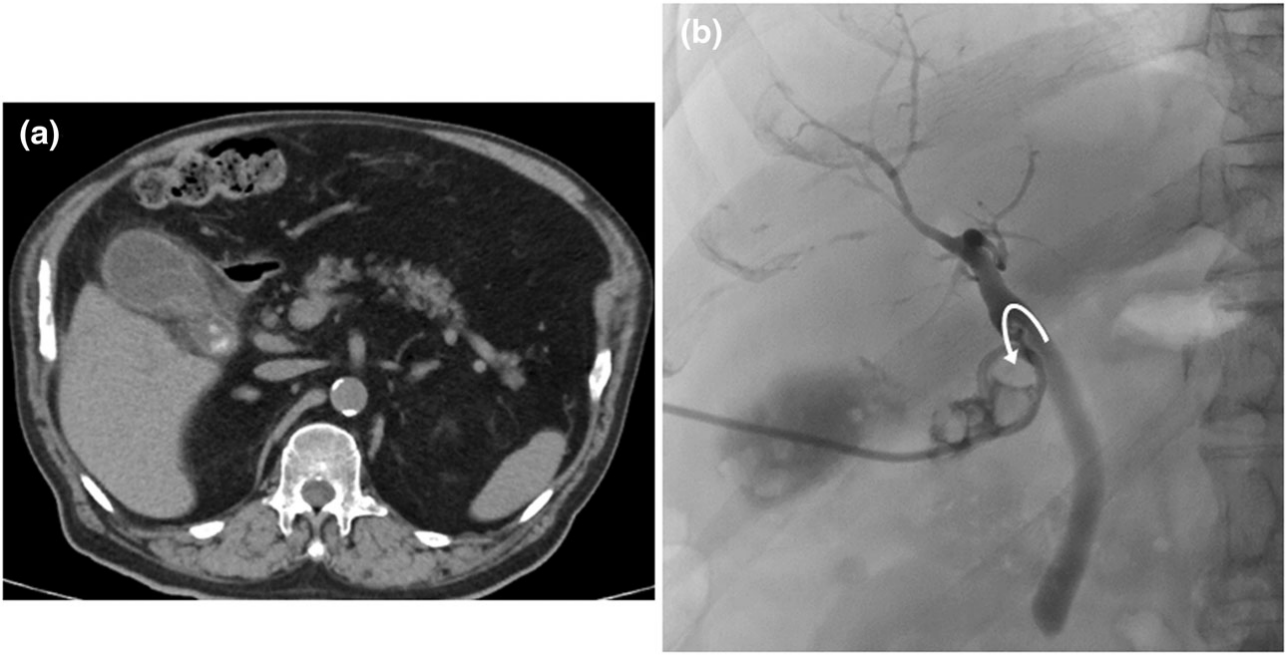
(a) Computed tomography scan showing cholecystitis with stones in the neck of the gallbladder. (b) Cholangiography during percutaneous transhepatic gallbladder drainage showing the cystic duct branching to the caudal side (arrow).
